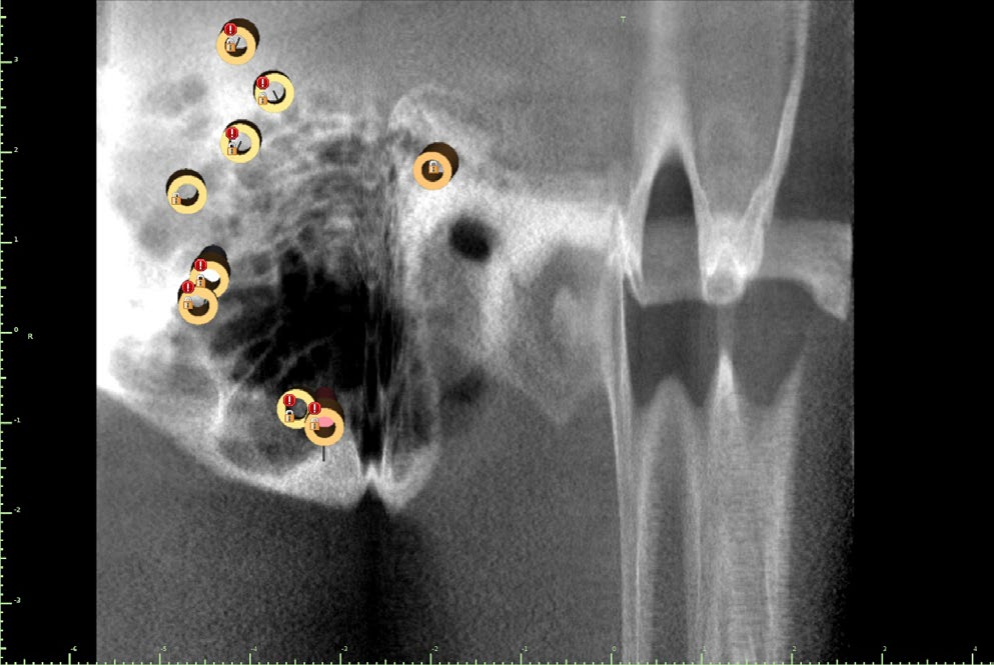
Axial CBCT image of prospective implant sites on right temporal bone, showing significantly pneumatized mastoid portion
